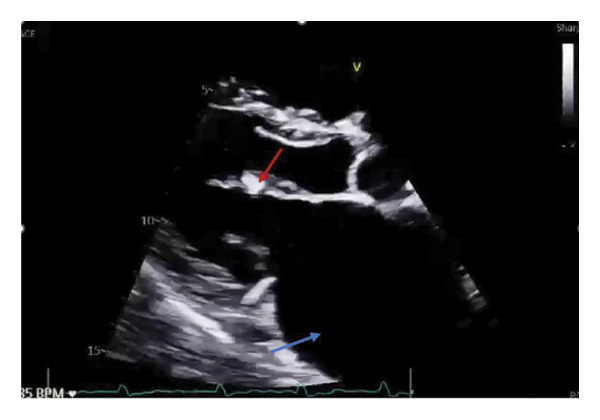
Transthoracic echocardiogram, parasternal long axis view. Note the thickened mitral leaflets (red arrow) and increased size of the left atrium (blue arrow).
